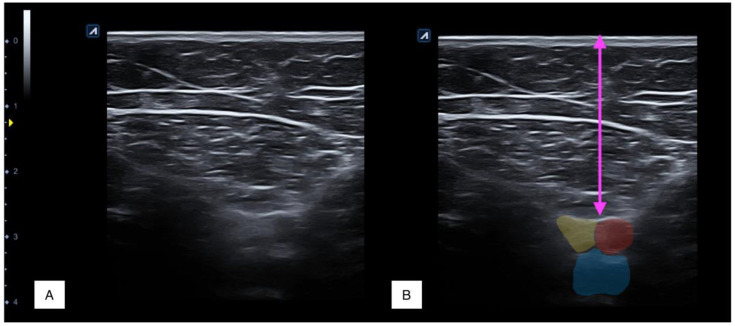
Ultrasound imaging of sartorius muscle at the proximal third (A). The pink arrow indicates the distance between the skin and the deep sartorius fascia limit. The femoral bundle is colored in yellow (nerve), red (artery), and blue (vein) (B).
